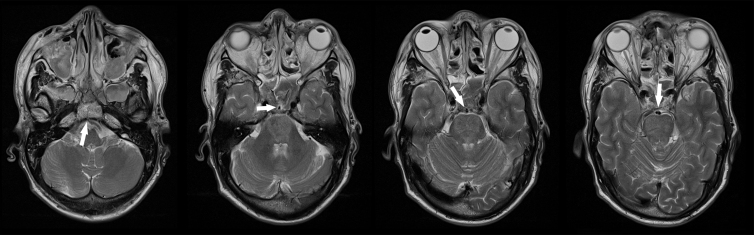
Axial magnetic resonance images. Sequential T2-weighted images (from caudal toward cranial) of the basilar artery pathway showing herniation of the artery into the clivus fracture and the trans-sinussoidal course through the left sphenoidal sinus.
